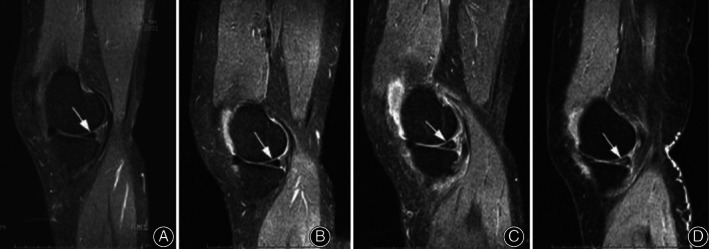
T2WI STIR for grading of meniscus injury. (A) Grade 0: Normal, MRI indicated the medial meniscus was integral, in smooth margin and in even and uniformed low signal (as shown by the arrow). (B) Grade I: The isolated dotted high signal indicated the meniscus articular surface margin was not invaded, medial meniscus was integral and in smooth margin (as shown by the arrow). (C) Grade II: The linear high signal indicated the meniscus articular surface margin was not invaded (as shown by the arrow). (D) Grade III: The high signal of medial meniscus indicated articular surface margin was invaded, accompanied with irregular meniscus (as shown by the arrow).
